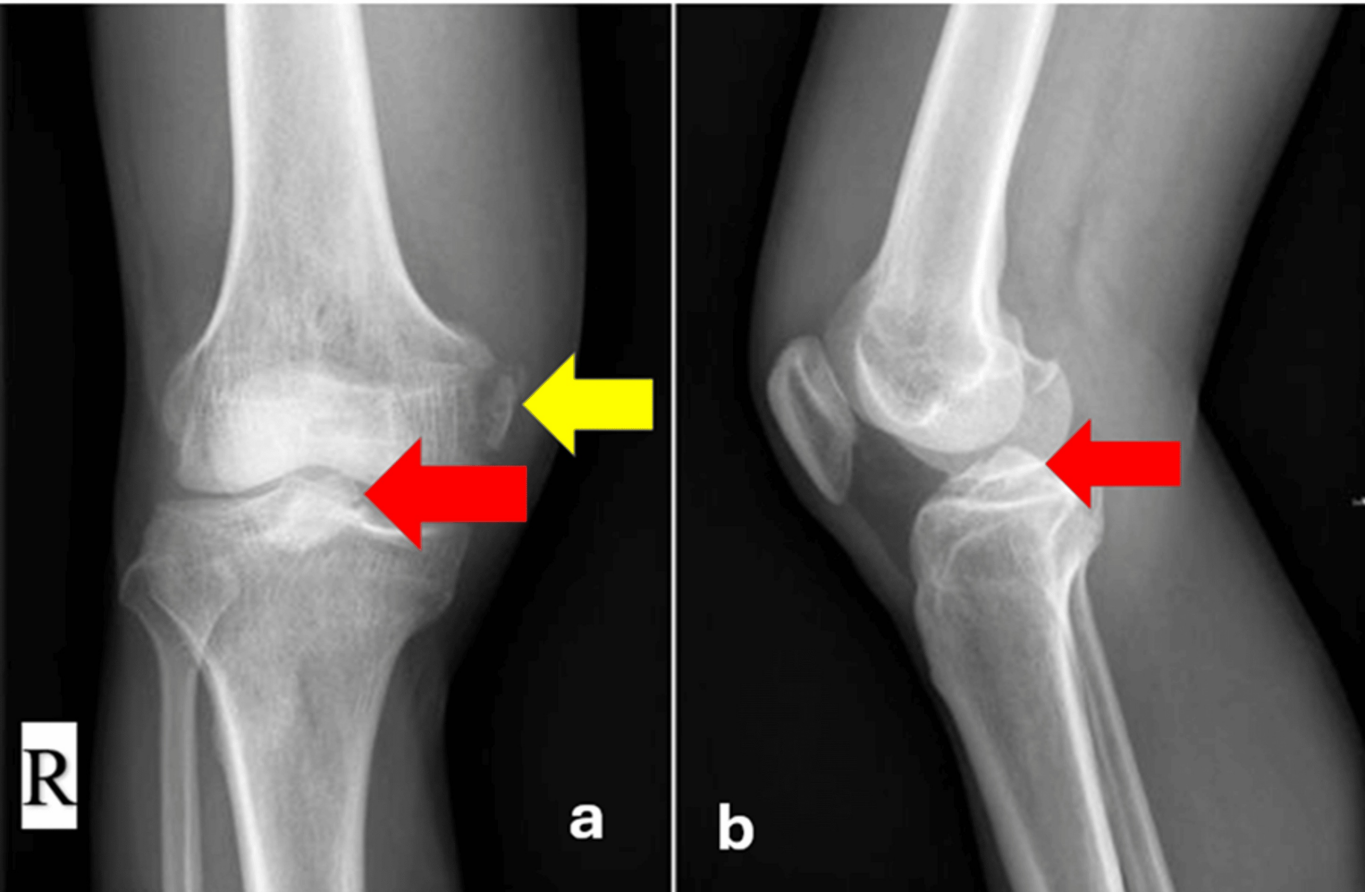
X-ray of the right knee in anteroposterior (a) and lateral (b) views. (a) Anteroposterior view shows avulsion of the anterior cruciate ligament (ACL) from the tibial plateau (red arrow) and avulsion of the medial collateral ligament (MCL) from the femoral condyle (yellow arrow).
